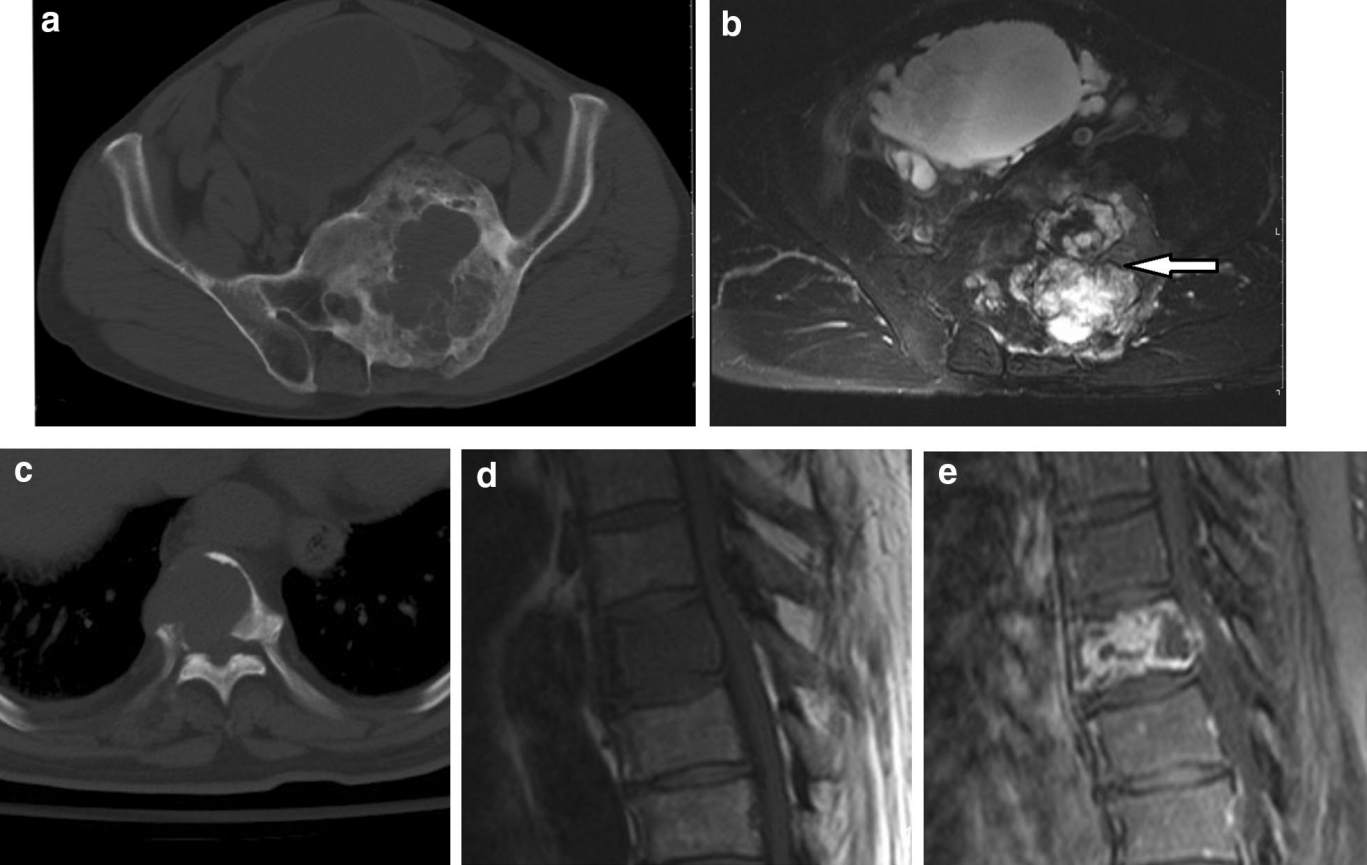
Case 14–15: Case 14, a Axial CT image demonstrates a large mixed lytic-sclerotic lesion, with a massive soft tissue mass confined to bone. b Axial fat-supressed T2-weighted MR image shows a high hetergeneous signal indicating cystic/necrosis components (*white arrow*) inside the lesion. Case 15, c Unenhanced CT image at bone window settings shows bone destruction with purely lytic appearance with discontinuous cortex and paraspinal mass. d, e Sagittal T1-weighted image and fat-suppressed T1-weighted postcontrast image show a heterogeneous hyperintensity and mild compression of the vertebral body
